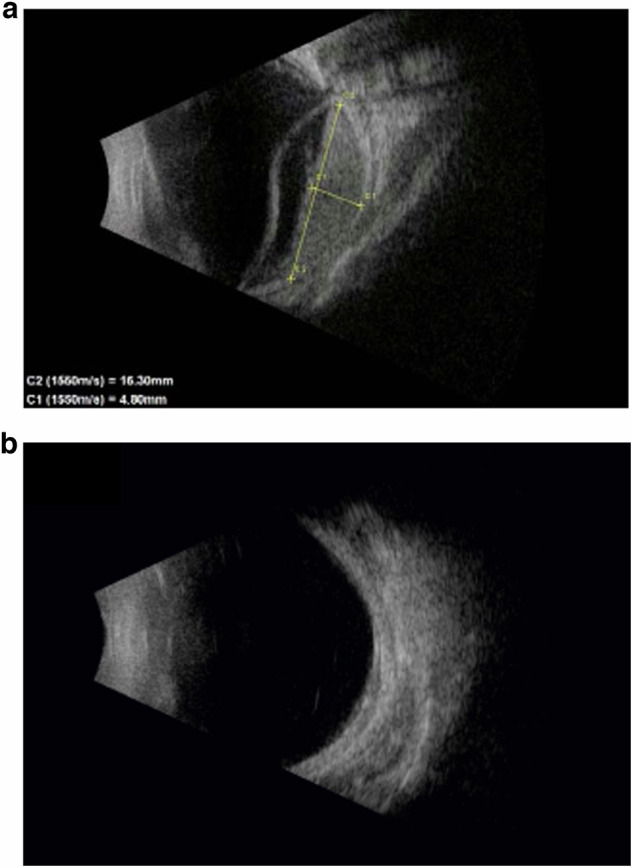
Ultrasound guided Ru 106 plaque brachytherapy for treatment of exudative retinal detachment in children with diffuse choroidal haemangioma. Pre-operative (a) and post-operative (b) B scan ultrasound images of an eye with diffuse choroidal haemangioma that underwent ultrasound-guided plaque brachytherapy showing tumour regression and resolution of exudative detachment after 7 months. (Case 1).
